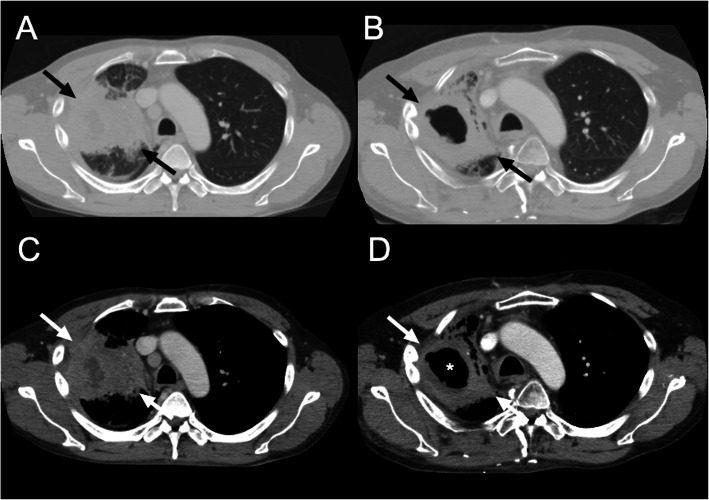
Comparison between RECIST and Lee’s criteria in NSCLC. A 51-year-old man was diagnosed with NSCLC. A Pretreatment CE axial CT scan in lung window shows a 92-mm-sized tumor, including both solid and GGO components (black arrows). B After targeted therapy with afatinib, posttreatment CE CT scan shows no significant decrease in tumor size (84 mm in diameter, 9 % reduction) (black arrows), suggesting stable disease according to RECIST 1.1. C According to Lee’s criteria, the size of the target lesion (white arrows) is measured on pretreatment CE axial CT by solid component alone (79 mm in diameter) on soft tissue window imaging. D After targeted therapy, the size of the target lesion is measured by including only soft-tissue tumor (white arrows) (77 mm in diameter) and excluding necrotic air cavitation (asterisk) (49 mm in diameter), thus the tumor size is 28mm (65 % reduction), suggesting good tumor response according to Lee’s criteria
